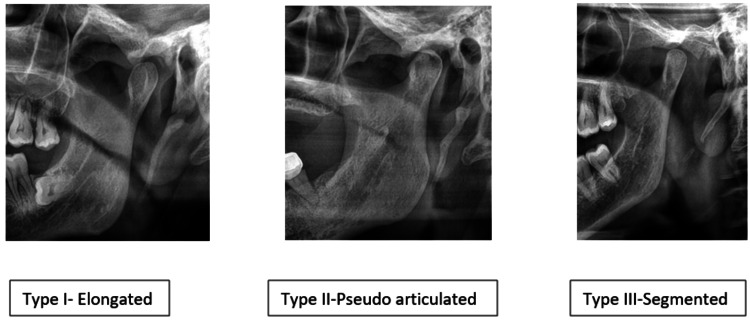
Elongation of styloid process in OSMF patients according to Langlais classification in OPG images. OSMF: oral submucous fibrosis; OPG: orthopantomogram.
